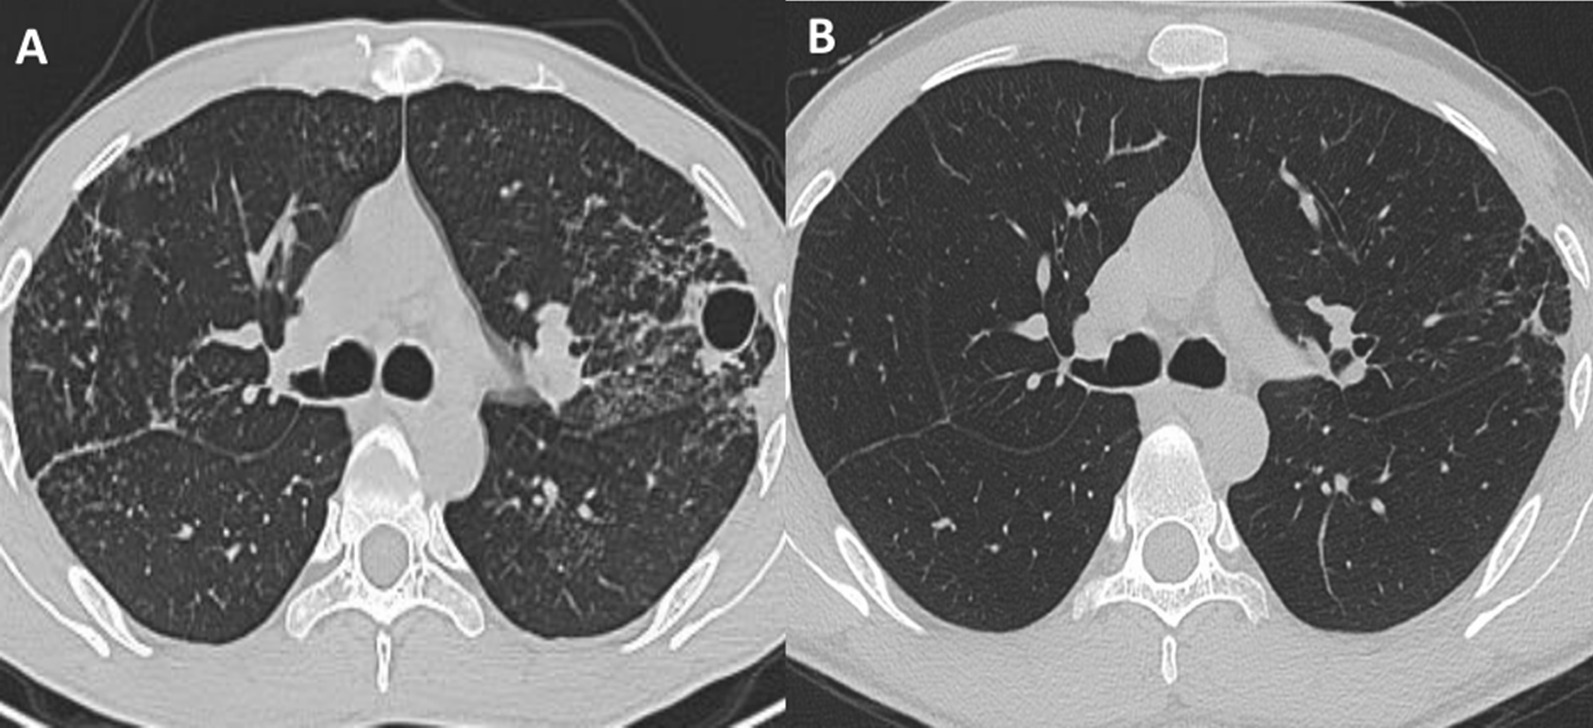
(A) Chest CT showed multiple infectious lesions in the upper lobe of the double lungs during the first hospitalization, which were absorbed obviously in the second hospitalization (B)
